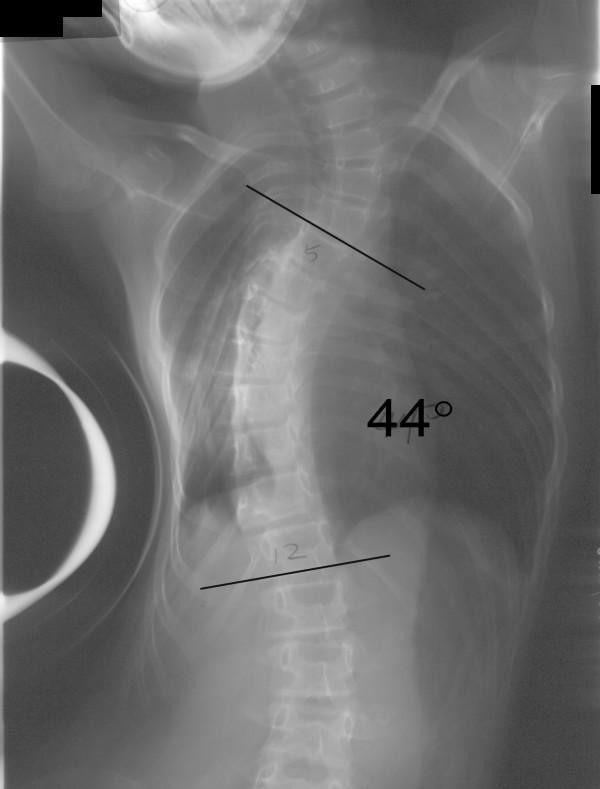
Fulcrum bending radiograph before anterior release.
